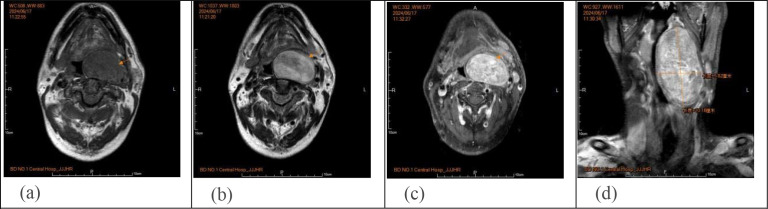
(a, b) MRI of the neck: Long T1 and T2 signal mass shadows can be seen in the left parapharyngeal space, with uneven signals, and T2 signal shadows, such as multiple plaques were seen in the space. The lesion was about 5.7×3.9×10.0cm in size, and the boundary was clear and smooth. (c, d) Enhanced MRI of the neck: Contrast-enhanced scan showed obvious uneven enhancement, the pharyngeal cavity was narrowed to the right, the left submandibular gland was compressed and moved outward, and the left common carotid artery and internal and external carotid artery were compressed and moved outward.
